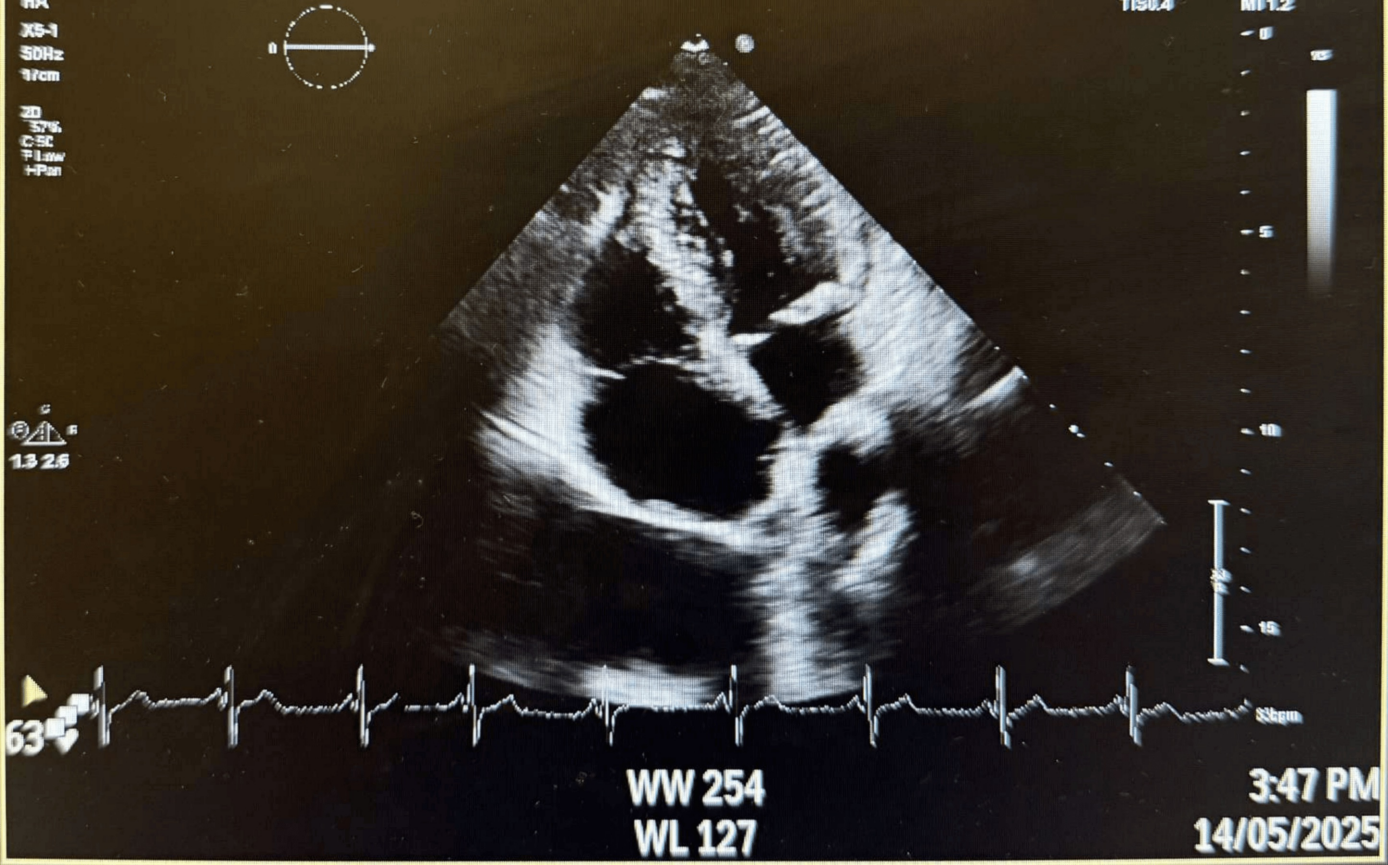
Bedside transthoracic echocardiography demonstrating preserved biventricular function with no intracardiac thrombus
